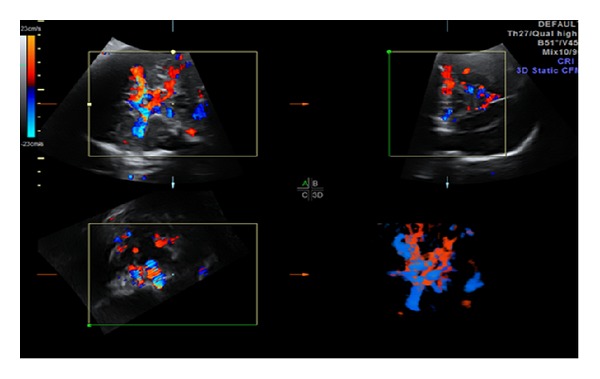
Increased brain vascularity seen on 2D and 3D ultrasound.
